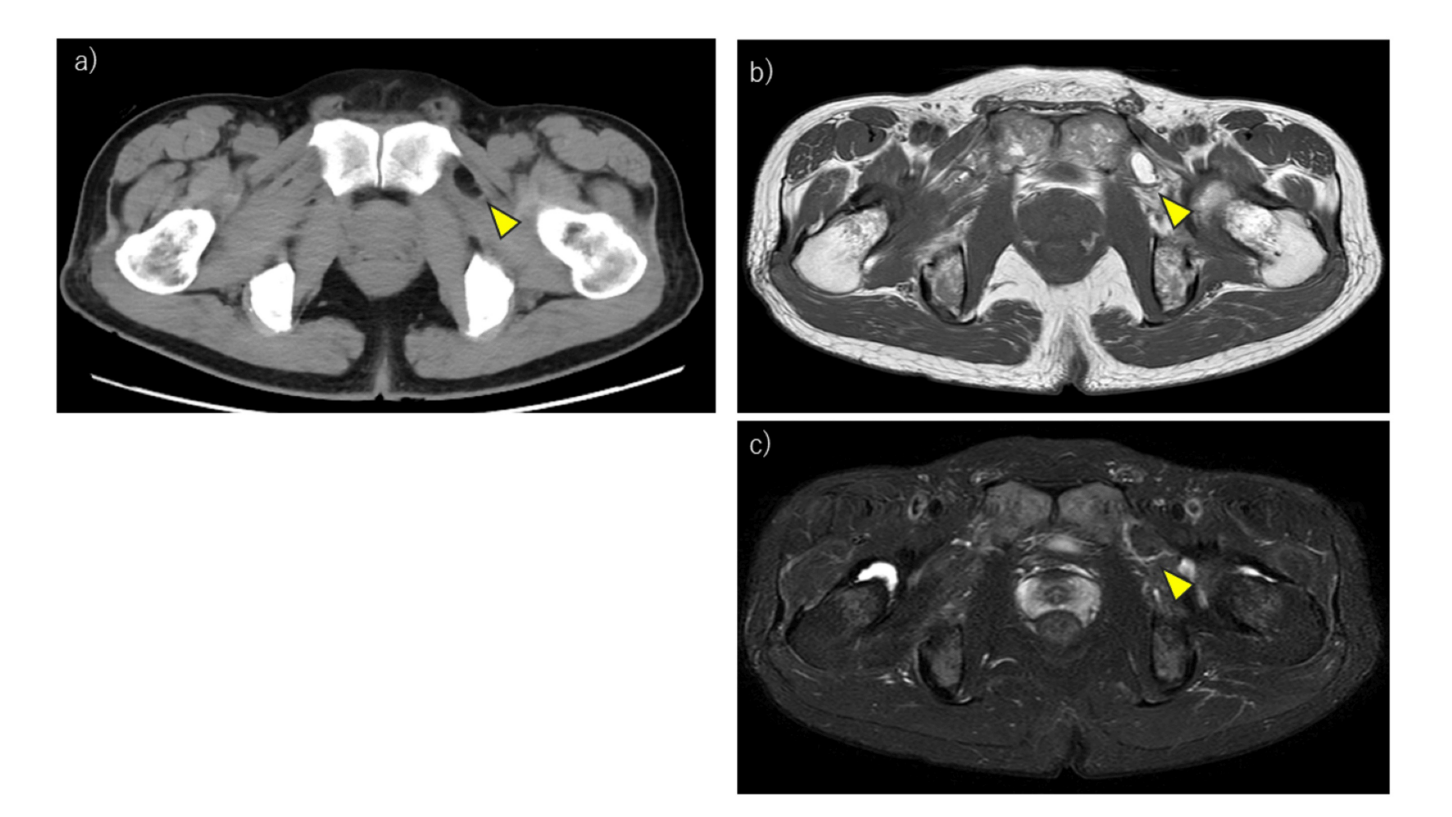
Preoperative imaging (a) CT showing fat herniation into the left obturator canal (yellow arrowhead). (b) MRI T2-weighted image showing high signal intensity in the corresponding area (yellow arrowhead). (c) Fat-suppressed MRI demonstrating signal suppression of the same lesion (yellow arrowhead).
